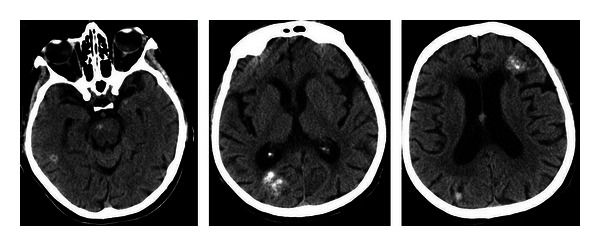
Neurocysticercosis—the nodular calcified stage. In these axial CT scan slices, multiple spontaneous hyperdense lesions are seen, some with pseudocystic aspects, with calcifications inside and without oedema or enhancement, located at the cortex/subcortical white matter border, in temporal, parietal, and frontal lobes. One pontine lesion is visible as well. Department of Neurology, Colentina Clinical Hospital brain imaging archive.
